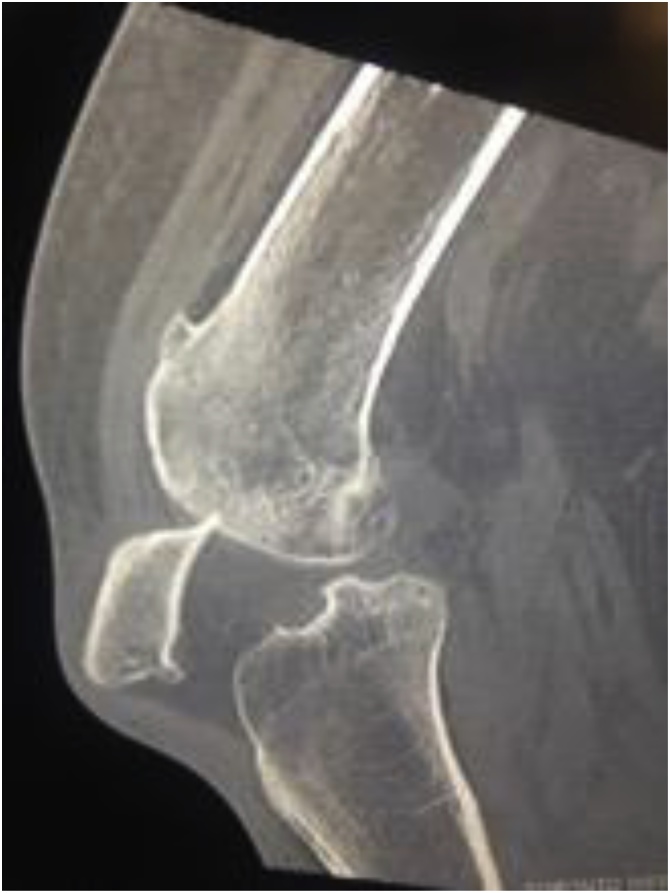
Left Knee CT (Sagittal).
